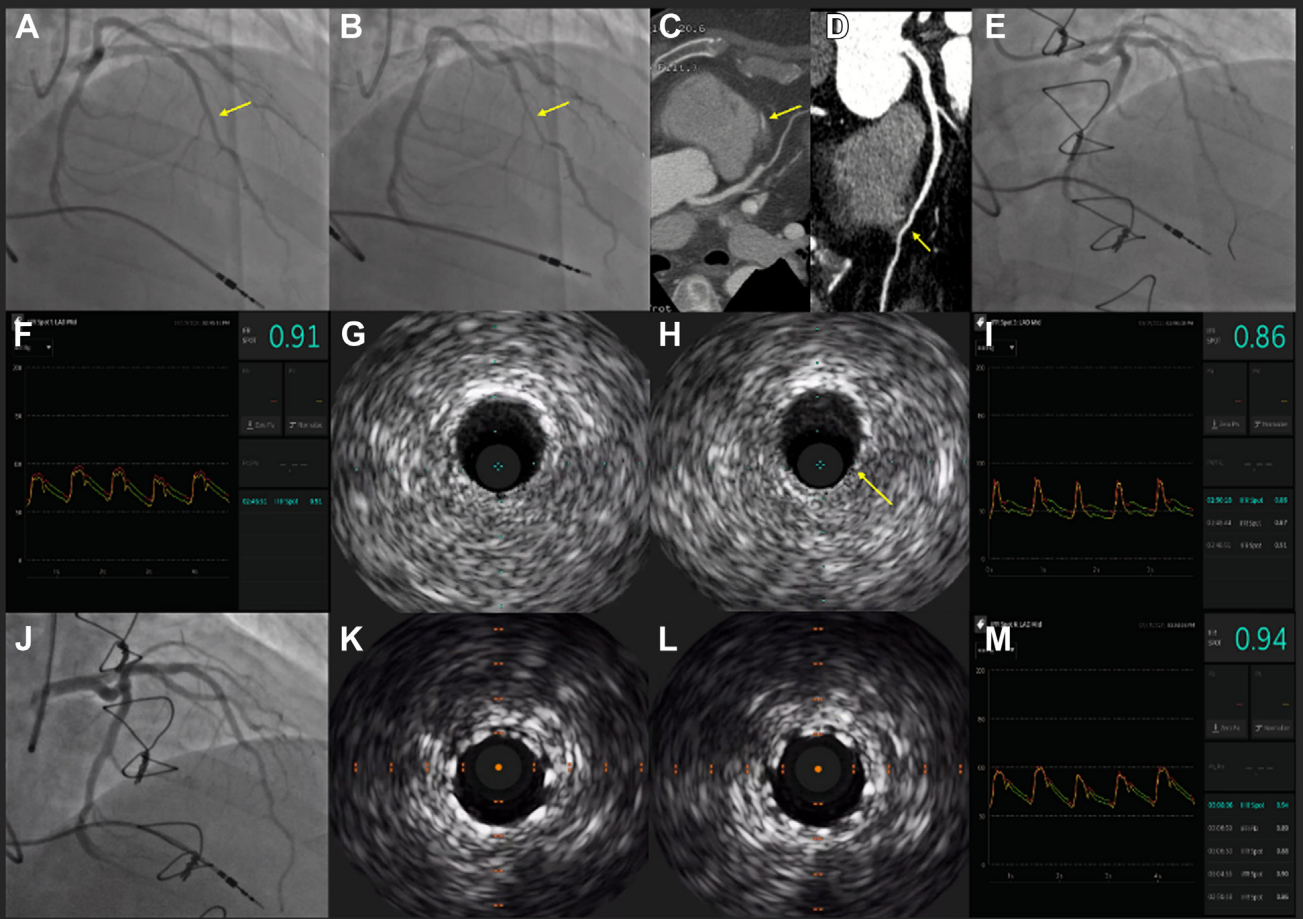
Multimodality assessment of a myocardial bridging segment. (A, B) Coronary angiography of a bridged segment (arrows) in the mid-LAD. (C) Coronary CTA showing intramyocardial course (arrow) of the mid LAD (preoperative). (D) Coronary CTA showing intramyocardial course (arrow) of the distal LAD (postoperative). (E) Coronary angiography of the mid-to-distal LAD without evidence of recurrent bridging. (F) iFR of the mid-to-distal LAD showing no ischemia at rest. (G-H) IVUS of the mid-to-distal LAD showing dynamic vessel compression (arrow) on 20.0 μg/kg/min of dobutamine. (I) iFR of the mid-to-distal LAD showing ischemia on dobutamine. (J) PCI of the mid-to-distal LAD with a 2.75 × 26.0-mm Resolute Onyx DES (Medtronic), postdilated to 3.0 mm proximally. (K-L) IVUS showing excellent stent apposition, expansion, and lack of dynamic vessel compression on 20.0 μg/kg/min of dobutamine. (M) iFR of the mid-to-distal LAD showing no further ischemia while on dobutamine. CTA, computed tomography angiogram; DES, drug-eluting stent; iFR, instantaneous wave-free ratio; IVUS, intravascular ultrasound; LAD, left anterior descending coronary artery; PCI, percutaneous coronary intervention.
